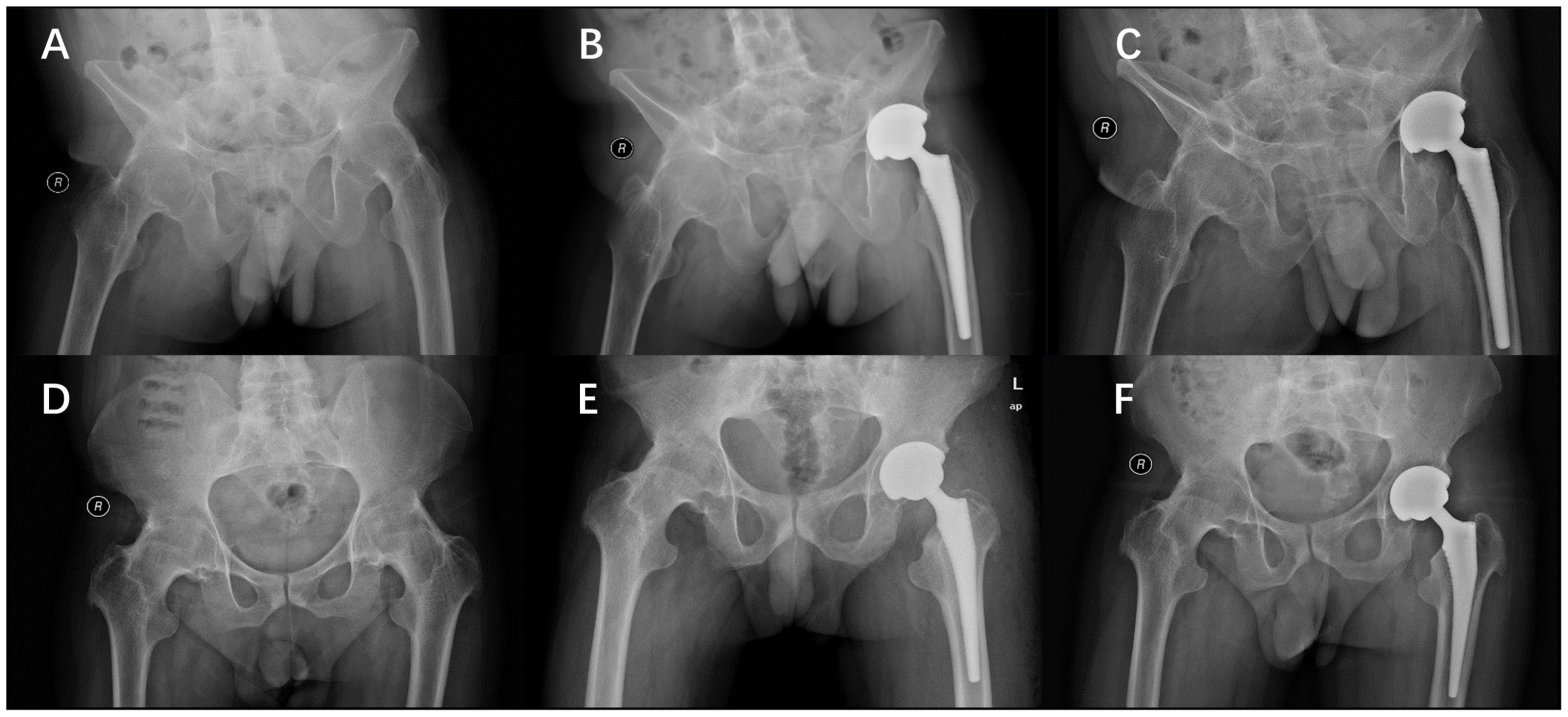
Conversion of extended hip fusion to DAA-THA in a 30-year-old male. Preoperative (A), 1 month (B) and 1 year postoperative (C) radiographs. Conversion of flexural hip fusion to DAA-THA in a 33-year-old male. Preoperative (D), 1 month (E) and 1 year postoperative (F) radiographs.
